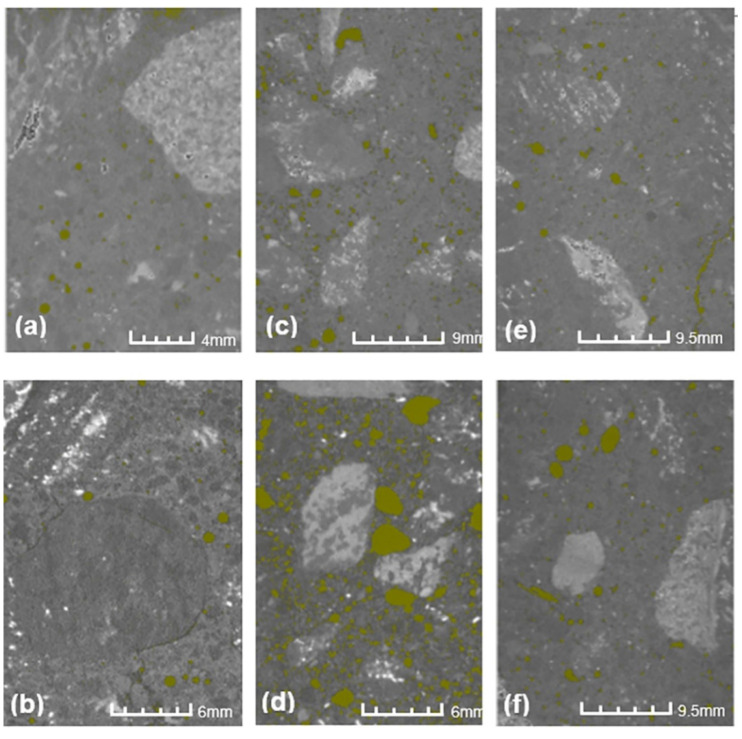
Air voids identification in computed tomography (CT) images: (a) CREF before heating; (b) CREF after heating; (c) CPP3 before heating; (d) CPP3 after heating; (e) CPW3 before heating; (f) CPW3 after heating.
